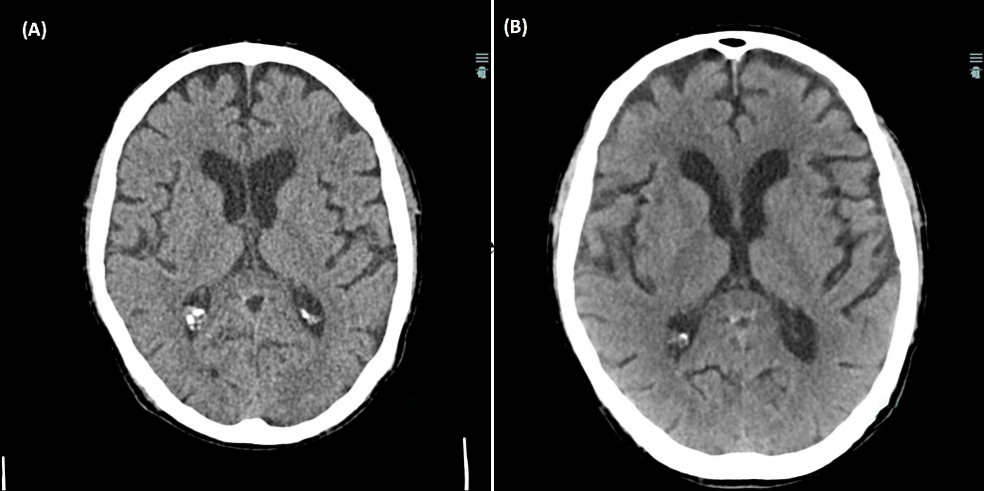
Computed tomography of the head without contrast from the 2017 admission (Panel A) and 2020 admission (Panel B).
